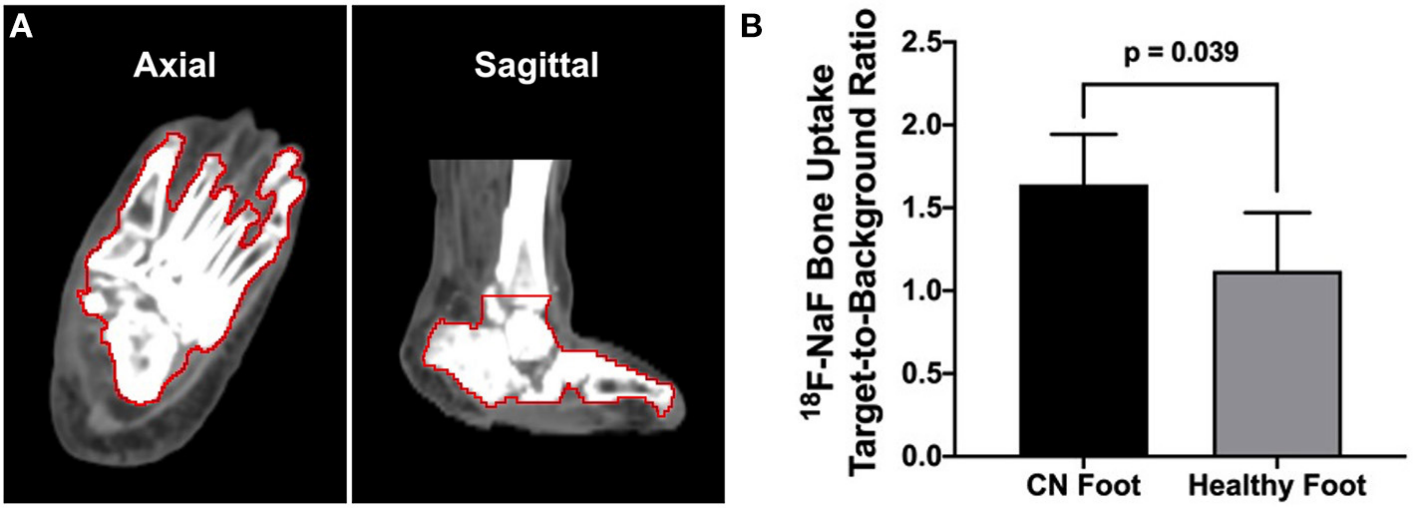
CT image-guided segmentation of the bones of the foot and quantitative ^18^F-NaF PET/CT image analysis. (A) Semiautomated segmentation of the bones of the foot and ankle (bone VOI outlined in red). (B) Quantitative analysis of PET/CT imaging demonstrated significantly higher bone uptake of ^18^F-NaF in the CN foot compared to the healthy non-CN foot. *N* = three patients. Values represent means ± SD.
